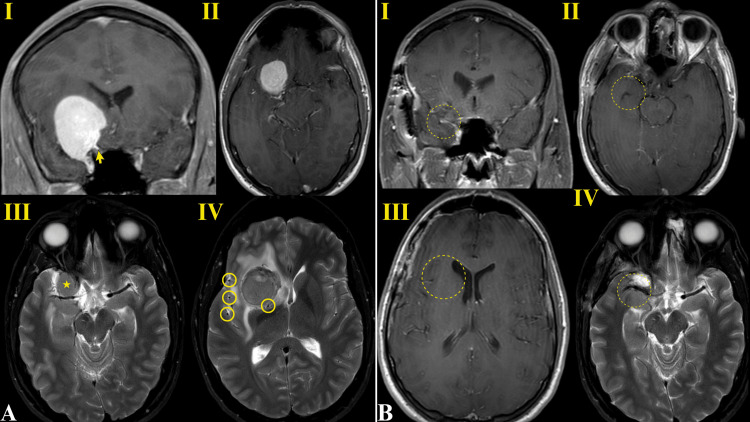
Preoperative (A) and postoperative (B) MRI findings The preoperative imaging is most notable for avid homogenous enhancement with a dural tail on T1 postgadolinium sequences identified by the yellow arrow in A_I_ and mild peripherally prominent flow voids on T2 sequences highlighted by the yellow circles in A_IV_, which are more consistent with typical meningioma appearance. A_II_ provides an axial view of the mass with the T1 sequence, and the star in A_III_ denotes the location of the mass at the level of the optic chiasm. The postoperative imaging was obtained three months following surgery and did not reveal any sign of residual or recurrent tumor. The approximated previous location of the tumor is displayed as a dashed circle in each panel in B_I-IV_. MRI: magnetic resonance imaging.
